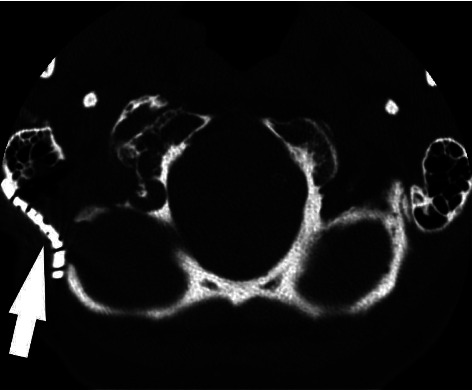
Axial CT image showing postoperative appearance. The hypoglossal canal was decompressed laterally via partial mastoidectomy, high cervical dissection, and partial resection of the occipital condyle. Metallic plating (arrow) covers the postoperative site.
